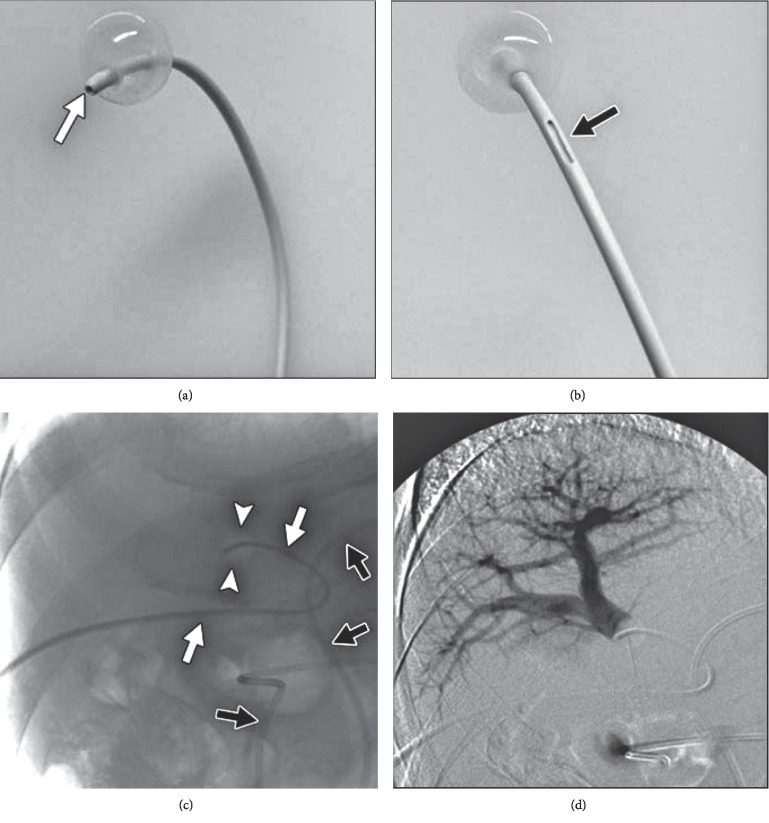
(a and b). Catheter used for portal vein embolization with absolute alcohol. This 5 French catheter has an end hole for ethanol administration, a side hole for contrast flushing, and an in-between balloon to prevent alcohol reflux (reprinted with permission from the American Journal of Roentgenology). (c). The triple lumen catheter (white arrow heads) with the balloon inflated is placed in the right portal vein. The black arrows refer to a nasobiliary drain. (d). Portography with the balloon inflated shows the anterior sectorial branch, which was embolized with absolute alcohol through the end hole (reprinted with permission from the *American Journal of Roentgenology*).
